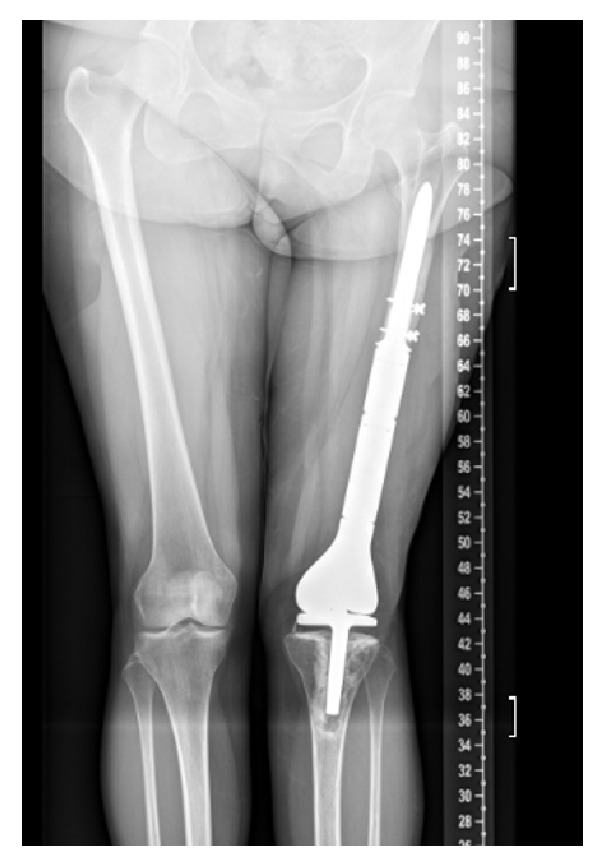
Postoperative AP radiograph with endoprosthesis reconstruction.
